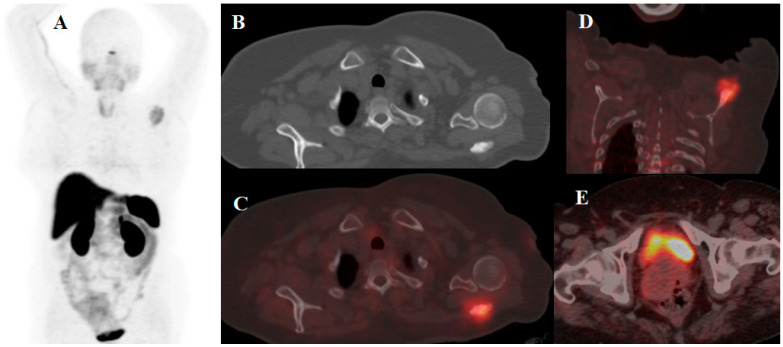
A 49-year-old woman with a history of NET of the cervix treated with local external beam radiotherapy referred for [^68^Ga]Ga-DOTA-TATE PET/CT scan due to left shoulder pain. (A) MIP image shows avid lesion in the in left shoulder area. Axial CT scan (B) and fused axial and (C) coronal (D) PET/CT images showed a sclerotic somatostatin receptor avid lesion in the body of the scapula, suggesting a NET metastasis. Interestingly, the primary tumor is in remission after local treatment (E).
